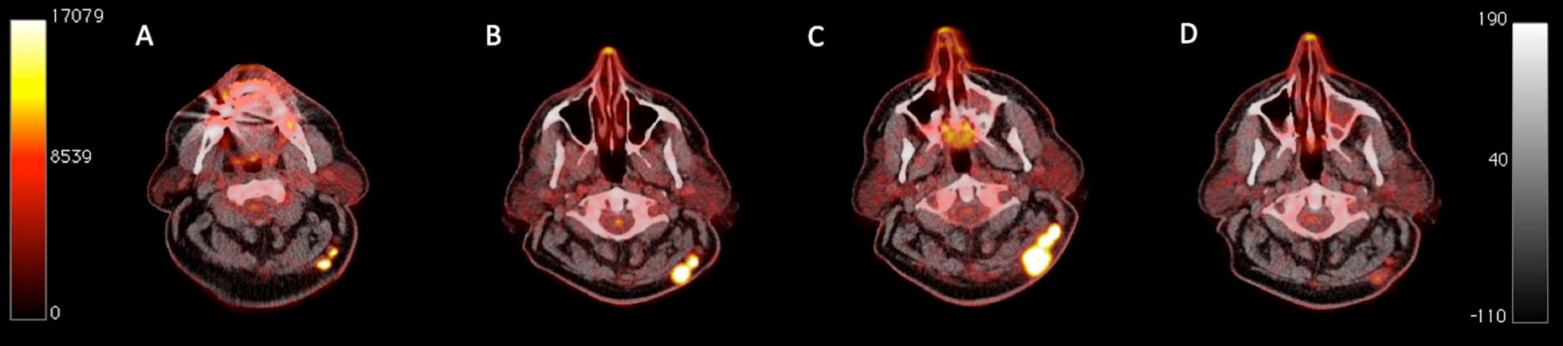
Transaxial PET/CT images at the cervical level of a 67-year-old melanoma patient undergoing immunotherapy with ipilimumab (a–d). Baseline PET/CT before commencement of therapy showed two hypermetabolic, subcutaneous metastases in the patient’s neck with SUV_max_ reaching 21.2 (a). After two cycles of ipilimumab, an increase in size and metabolism of the metastases was observed (SUV_max_ 28.8), leading to uPMD according to EORTC (b). The patient received two more cycles of ipilimumab and was re-examined with PET/CT, which demonstrated persistence of the hypermetabolic disease (SUV_max_ 25.5) and a further increase in lesions’ size (c). No further treatment was administered and, 4 months later, PET/CT demonstrated a marked morphologic and metabolic (SUV_max_ 4.2) remission of the lesions (d). Similar to the patient in Fig. 2, the application of PERCIMT classified the patient as SMD, thus tackling the phenomenon of pseudoprogression. At last follow-up the patient was still alive having reached an OS of 66.7 months
